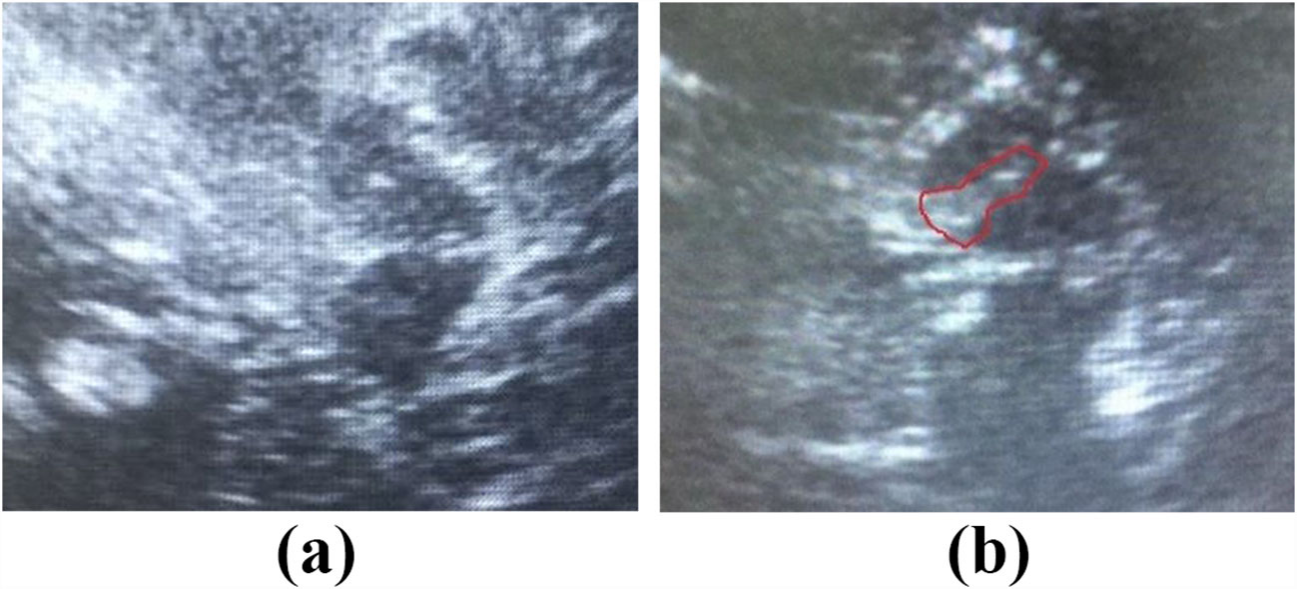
Sonographic images of the mesencephalic brainstem in a healthy control (a) and a patient with Parkinson’s disease (b). The butterfly-shaped mesencephalic brainstem was surrounded by the hyperechogenic basal cisterns. The patient with Parkinson’s disease exhibited hyperechogenic signals encircled by lines at both sides of SN, which were not seen in the control
